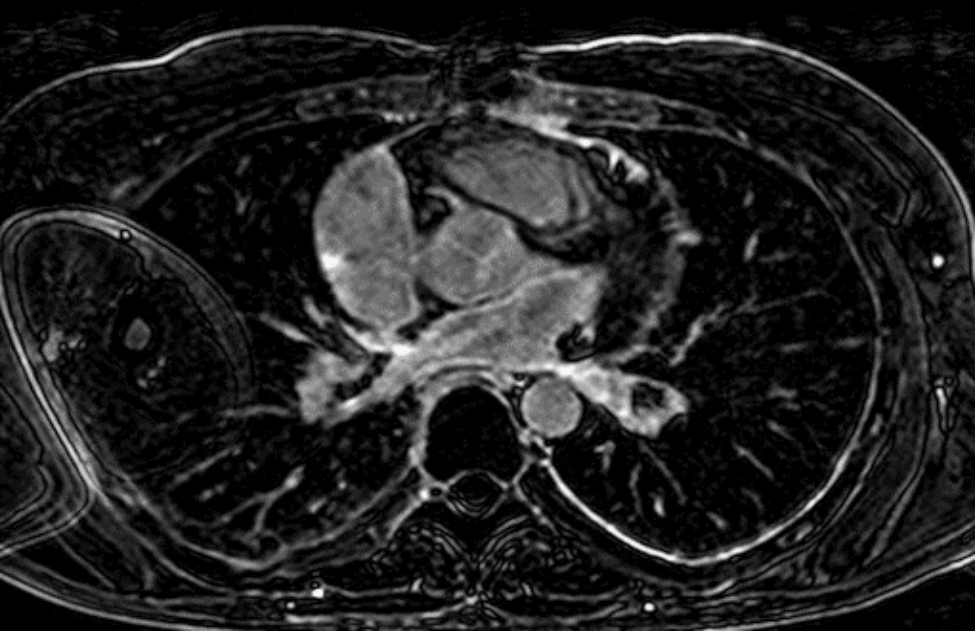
Cardiac 3D late Gadolinium magnetic resonance imaging showing an extremely small left atrium with extensive fibrosis
